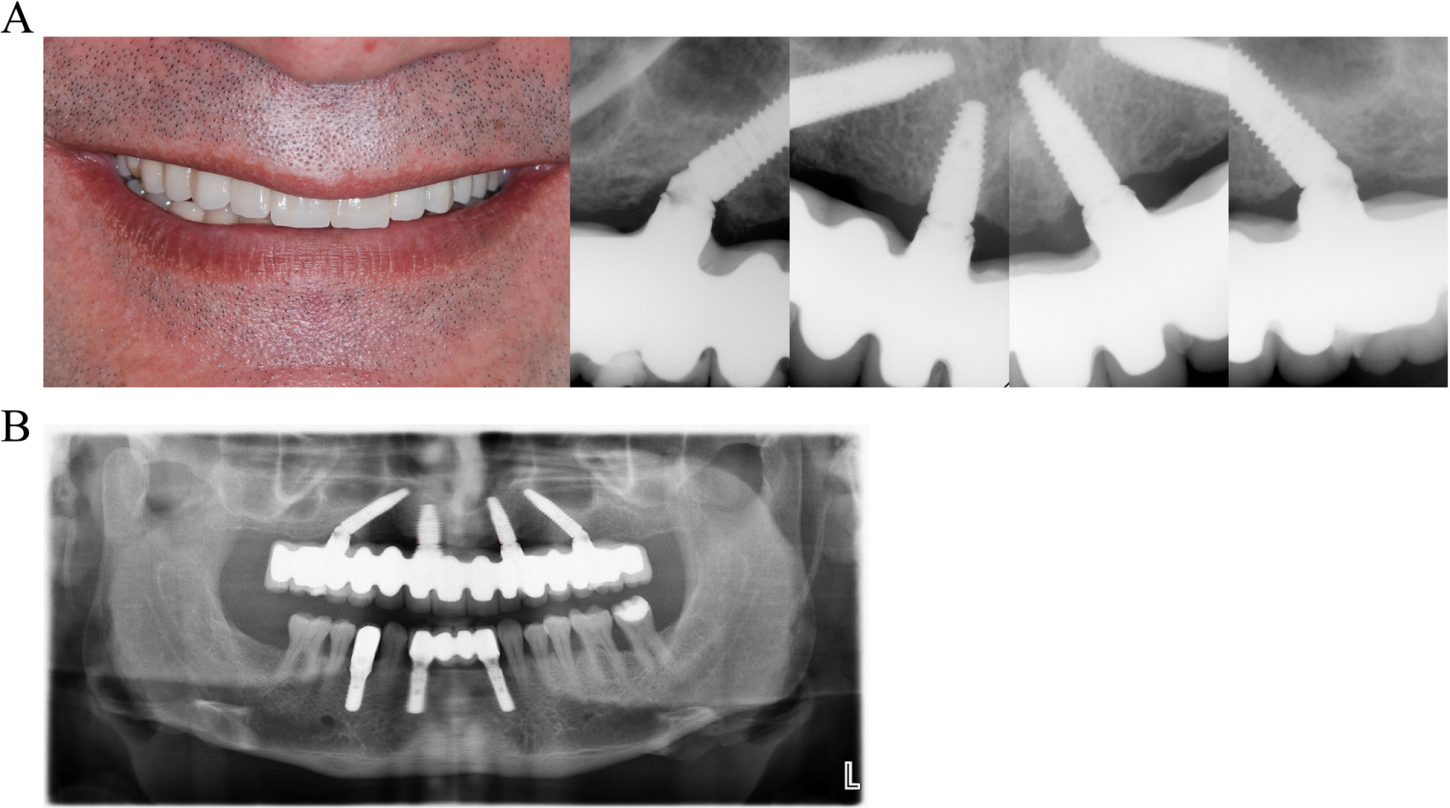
A view of the final ceramic prosthetic reconstruction (left) and periapical radiographs (right) of the case at 42 months of follow-up (a). The OPG view at the 42 months (b)
